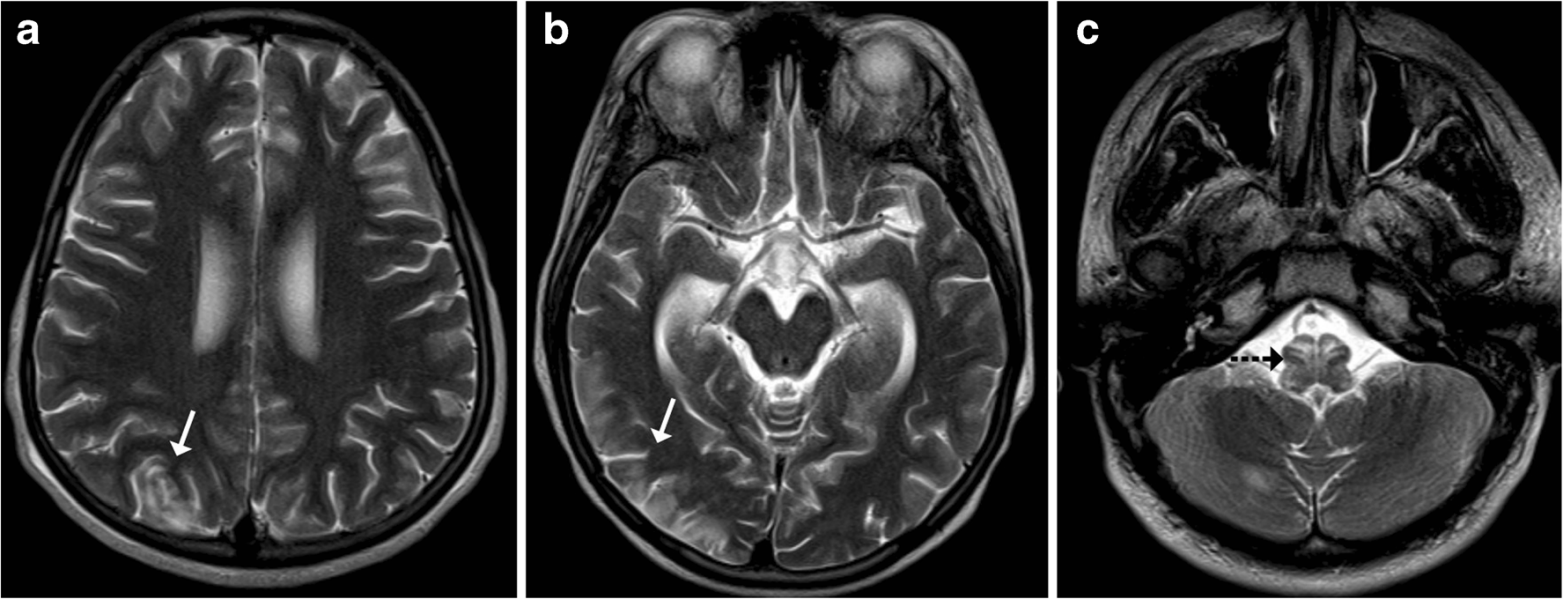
A 12-year-old patient with chronic renal failure and seizures: a–c Axial T2-weighted images reveal increased signal intensity in the right parieto-occipital cortex (*arrow*) with similar signal intensity at the cervicomedullary junction (*dotted black arrow*)
